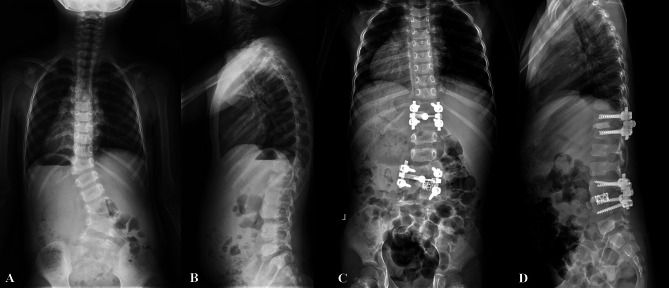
A case of mixed segmented hemivertebra with concomitant cardiac abnormalities A 4/F, diagnosed with congenital scoliosis, she had a fully segmented hemivertebra at T12 segment and partially segmented hemivertebra at L3/4 segment (a, b). Her ultrasonic cardiography result showed mitral valve redundancy and prolapse. And she underwent a 2-staged surgery, the X-ray showed hemivertebra at T12 and L3/4 was totally resected (c, d)
